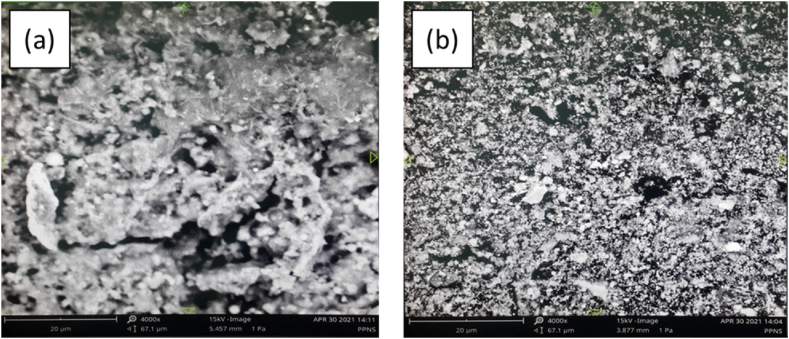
Micro photo of the substrates of a) WT, b) CTA.
